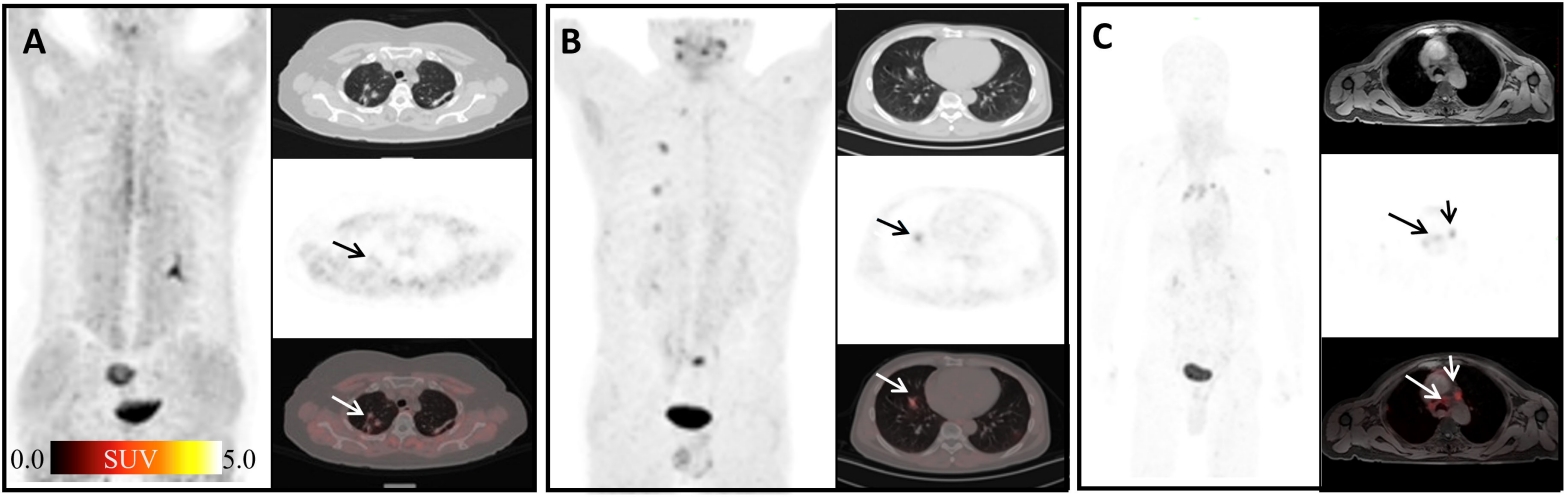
Physiological uptake of ^68^Ga-FAPI-04. (A) A 64-year-old female patient with gastric cancer 1 year after surgery; the arrows show organized pneumonia in the upper lobes of right lung with SUV_max_ of 1.76. (B) A 60-year-old male patient after liver cancer surgery; the arrows indicate the inflammatory lesion in the middle lobe of the right lung with SUV_max_ 3.24. (C) A 50-year-old male patient with weight loss of 10 kg in recent 6 months; arrows show mediastinal inflammatory lymph nodes with SUV_max_ of 4.91.
